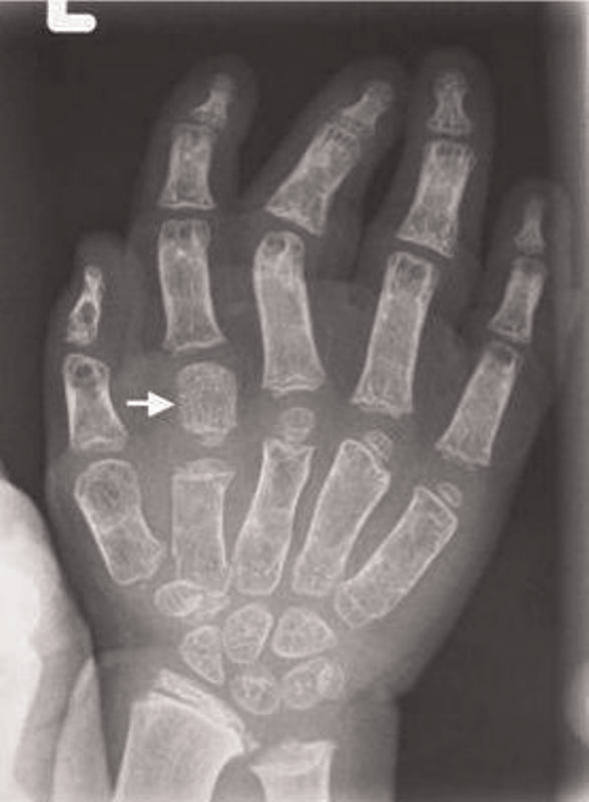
A-P radiograph of the hand showed multiple carpal ossification centres equivalent to bone age of 7 years 4 months. Supernumerary phalange characteristically situated between the metacarpal and proximal phalanx of the index finger, and existence of metaphyseal enlargement and pseudoepiphysis (arrow) were noted as well.
